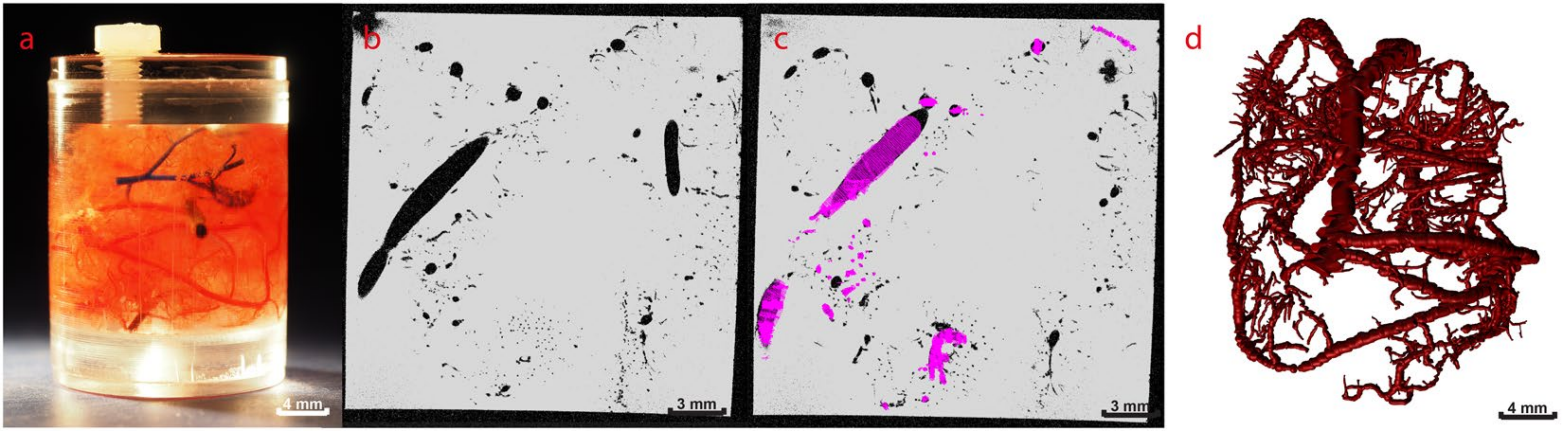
Small section of the human cerebral cast scanned with 9.4T MRI. (a) The cast section embedded in gel in a small plastic container, (b) Single slice from MRI (30 µm isotropic resolution), (c) Segmented arteries on single slice as displayed in Simple Neurite Tracer. (d) 3D visualisation of all reconstructed arteries.
